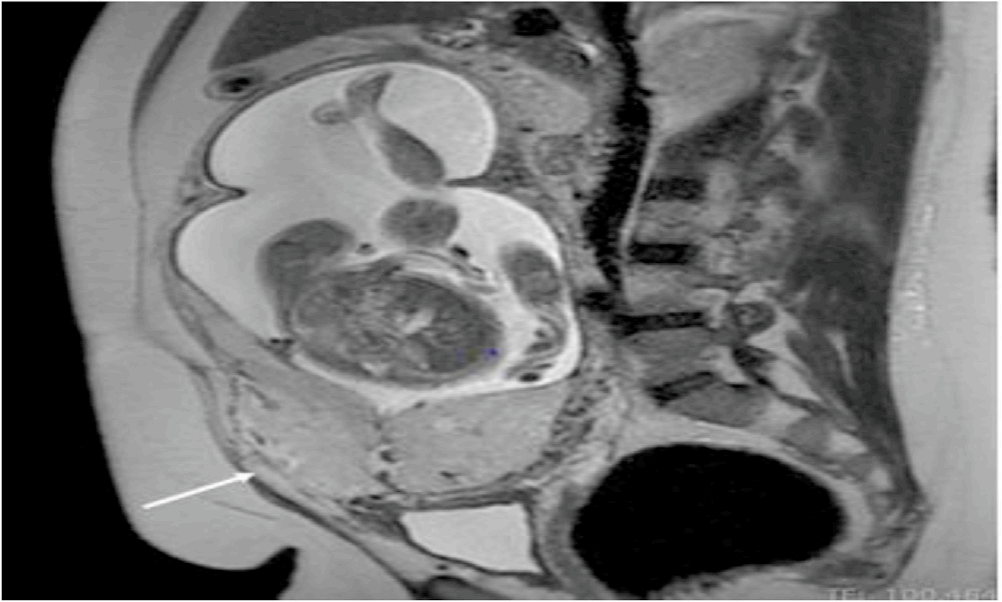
MRI sagittal T2-weighted image showing a completely covering placenta previa, loss of continuity in the placental-myometrial interface at the isthmic level with anterior bulging (arrow).
